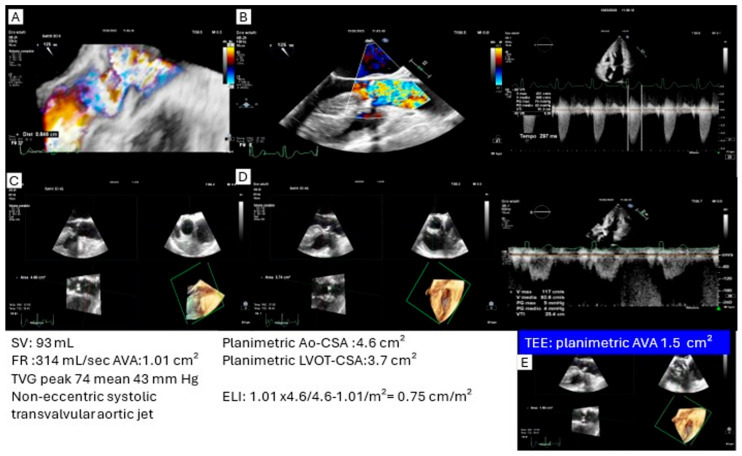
Case of an asymptomatic patient with high transvalvular gradients and discordant AVA (>1 cm^2^). TEE planimetry demonstrated an AVA of 1.5 cm^2^, while the calculated ELI (using direct measurements of aortic and LVOT CSA) was 0.75 cm^2^/m^2^, consistent with functionally non-severe AS. The high gradients were related to a high-flow state with marked pressure recovery due to a small ascending aorta and a non-eccentric transvalvular jet. ((A–E) transesophageal images of transvalvular aortic jet (A): 3d color flow mapping; (B): 2d color flow mapping), cross-sectional area left ventricular outflow tract (C) and cross-sectional aortic area at sino-tubular junction.
